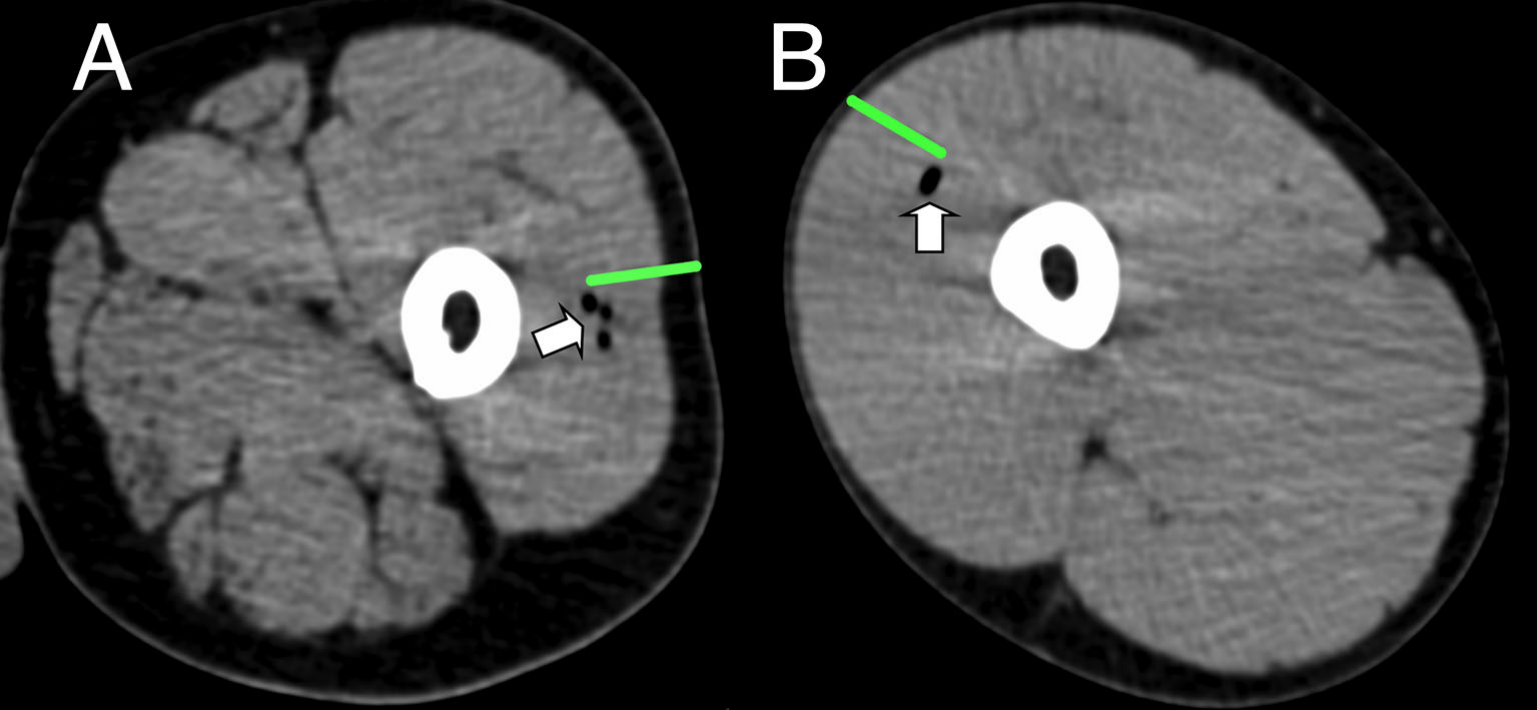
Representative CT imaging of intramuscular immunization site 3 h post injection. Transient inclusions (white arrows) visible at site of immunization with ATIV (A) and 4CMenB (B). Green bars indicate length of immunization needle, actual injection track unknown.
